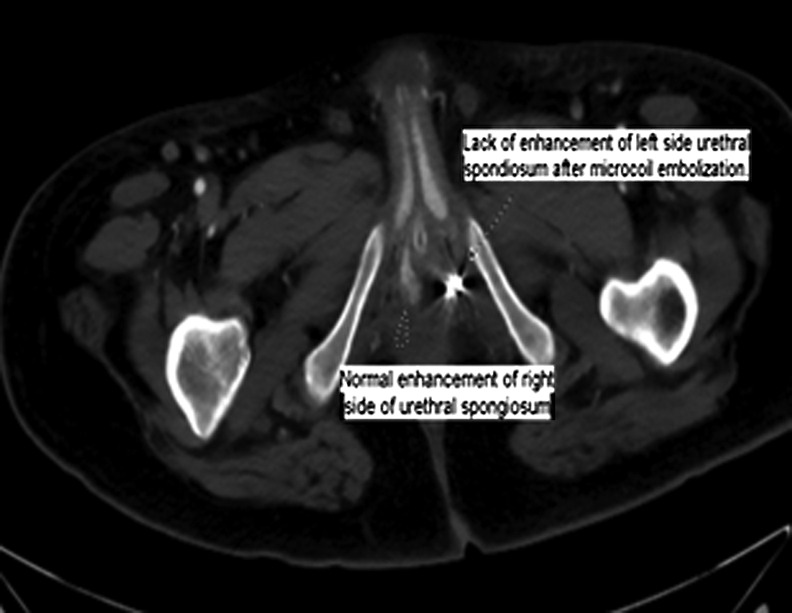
CT angiography demonstrates decreased enhancement of the left spongiosal tissue compared with the right spongiosal tissue after selective angioembolization.
